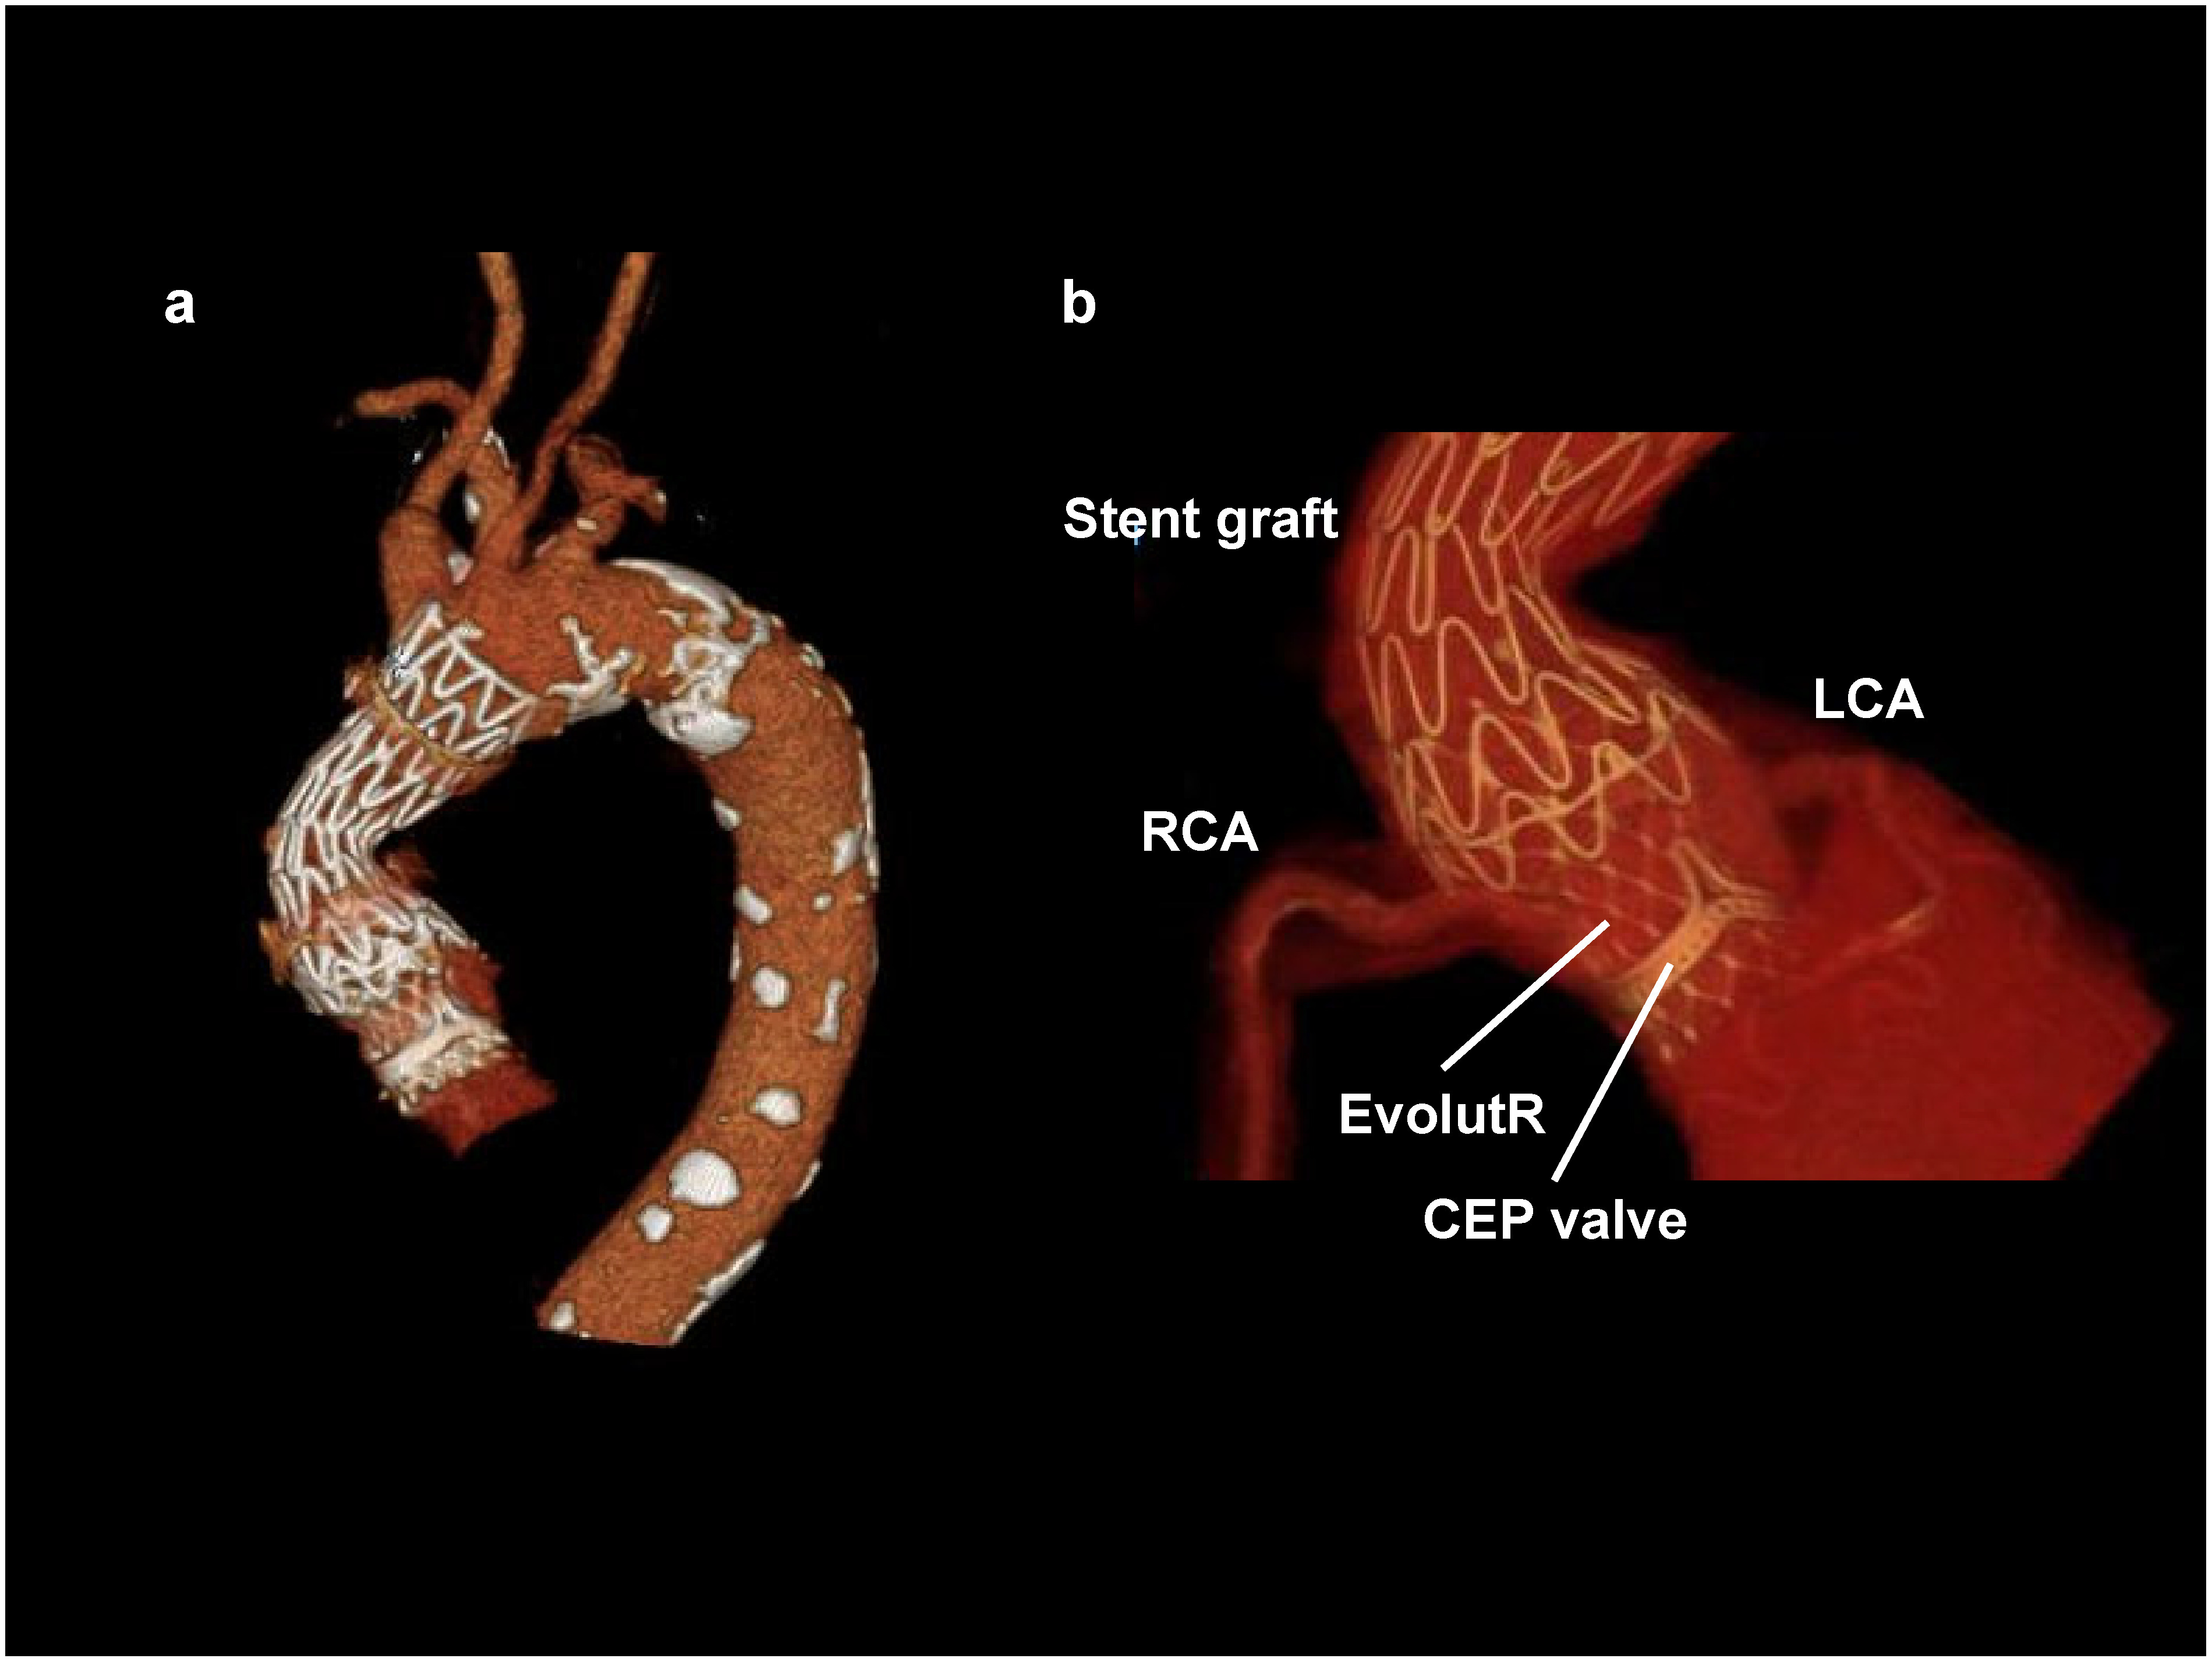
Fig. 3 Postoperative computed tomography showing the appropriately positioned aortic prosthetic valve and the stent graft in the ascending aorta with the patent (a) supra-aortic arch vessels and (b) coronary arteries.
